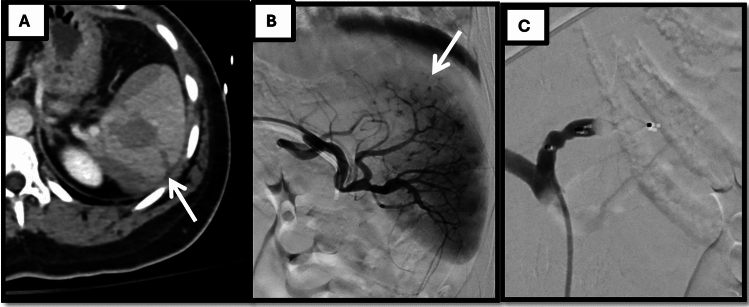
A Axial contrast-enhanced CT (CECT) of the abdomen in a 36-year-old female status post motor vehicle accident shows splenic laceration and perisplenic hematoma (arrow). B Selective splenic arteriogram demonstrates a tortuous splenic artery with multiple pseudoaneurysms within the splenic parenchyma (arrow) C Post-embolization digital subtraction angiogram (DSA) with LOBO-7 shows successful proximal splenic artery occlusion
